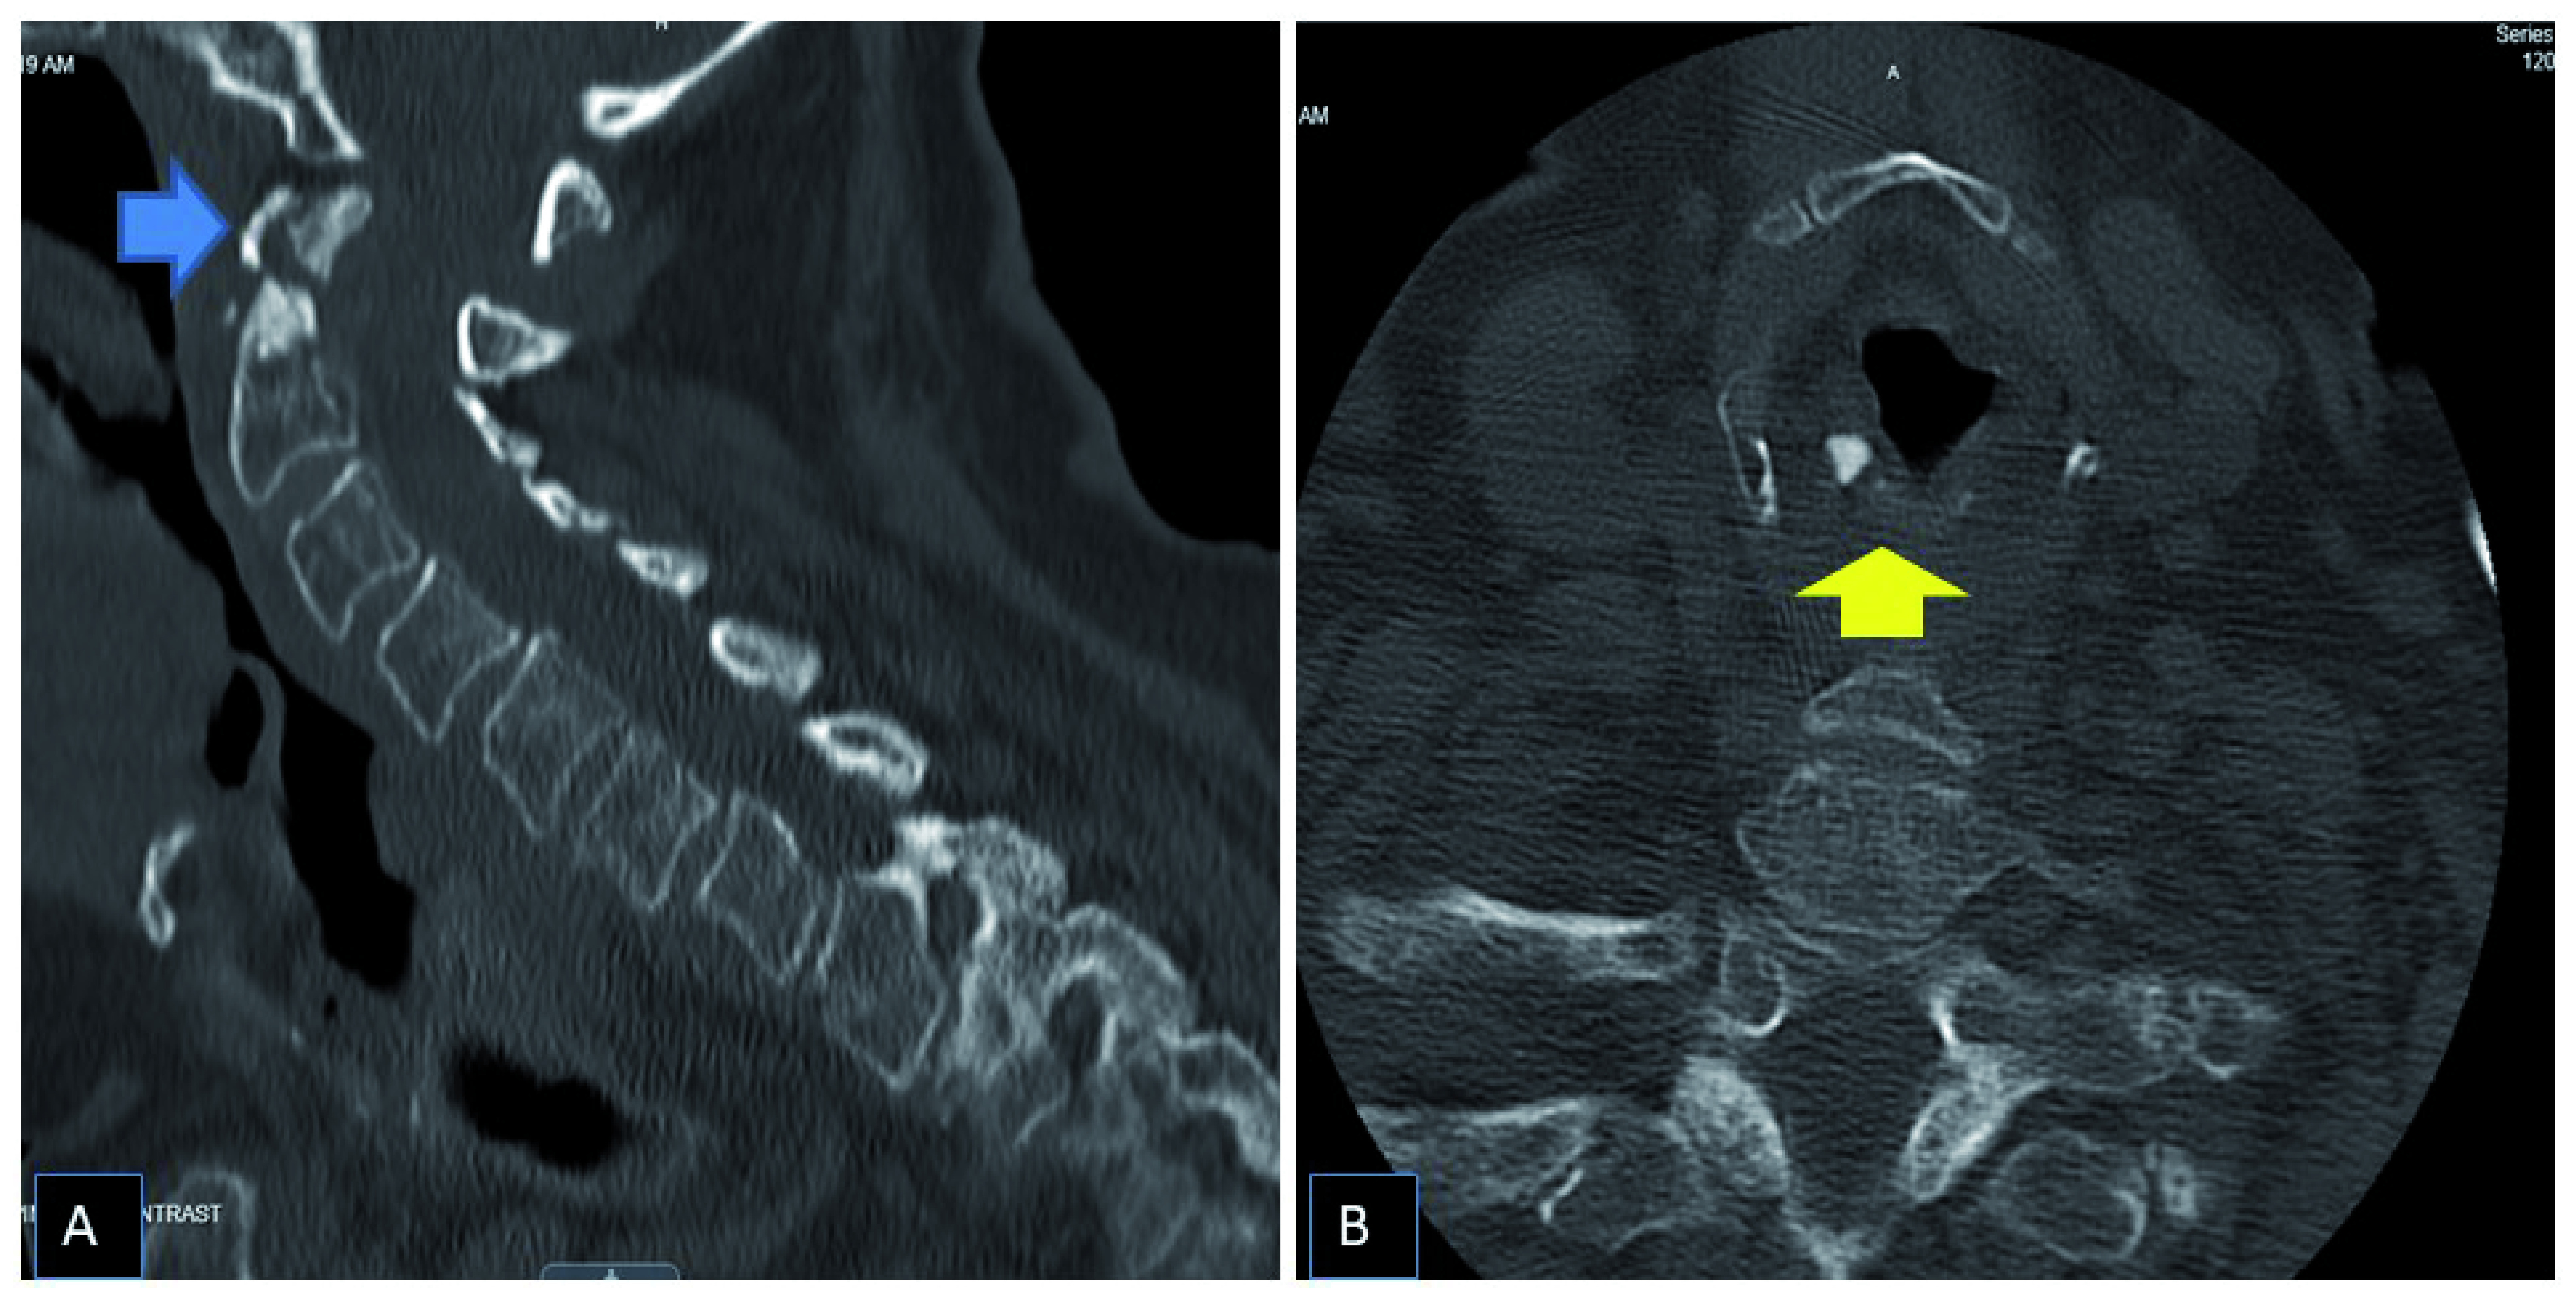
CT scan of the cervical neck showing Type II odontoid fracture (blue arrow) and sclerosis of the arytenoid cartilage (yellow arrow).
